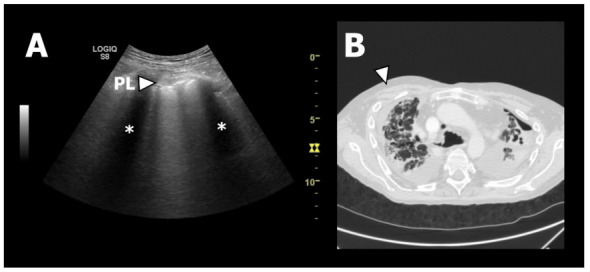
LUS and HRCT findings from a patient with RAS. (A): Lung ultrasound image from zone R1. At the top of the chest wall structures can be seen as a grey area. Just below the chest wall the pleural line (PL) can be seen as a horizontal white line. It has an abnormal appearance since it is thickened and fragmented. The two black areas (*) is caused by “shadowing” due to the ultrasound waves being absorbed and reflected by the ribs. (B): Axial HRCT image of the upper lobes showing fibrotic pleural and septal thickening consistent with PPFE. The white arrow corresponds to LUS zone R1 presented in A.
